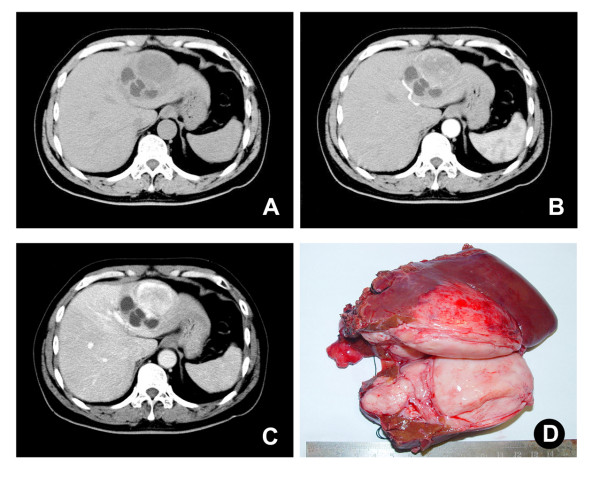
Single mass in the left hepatic lobe. (A) CT scan demonstrated a mass (9.0 × 6.2cm) in left hepatic lobe. (B) and (C) Contrast enhancement in arterial and portal phases was found in the mass. (D) Grossly, a large, gray-white, lobulated, well-circumscribed, partially encapsulated mass was removed after hepatoectomy.
